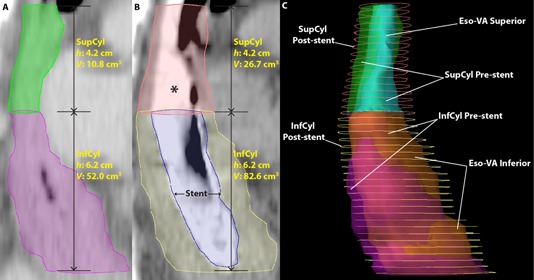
Volume approximation structures. CT sagittal image pre‐stent (a) showing SupCyl (green) and InfCyl (magenta), with height (h) and volume (V) shown. CT sagittal image of same patient (b) after self‐expanding stent (SES: blue) placement showing SupCyl (pink) and InfCyl (yellow), with height (h) and volume (V) shown. Both the SupCyl and InfCyl post‐stent volumes are larger than the pre‐stent volumes due to SES‐induced esophageal expansion. The * denotes contrast media in the esophagus. The proximal end of the stent (blue) ends at the top of InfCyl. Right lateral 3D reconstruction (c) showing the differences in volumes between the pre‐stent, post‐stent, and VA structures. The post‐stent volumes (contours, SupCyl: pink, InfCyl: yellow) are visibly larger than the pre‐stent volumes (transparent, SupCyl: green, InfCyl: magenta) and the VA structures (Eso‐VA Superior: cyan, Eso‐VA Inferior: orange) more closely approximate pre‐stent volumes.
